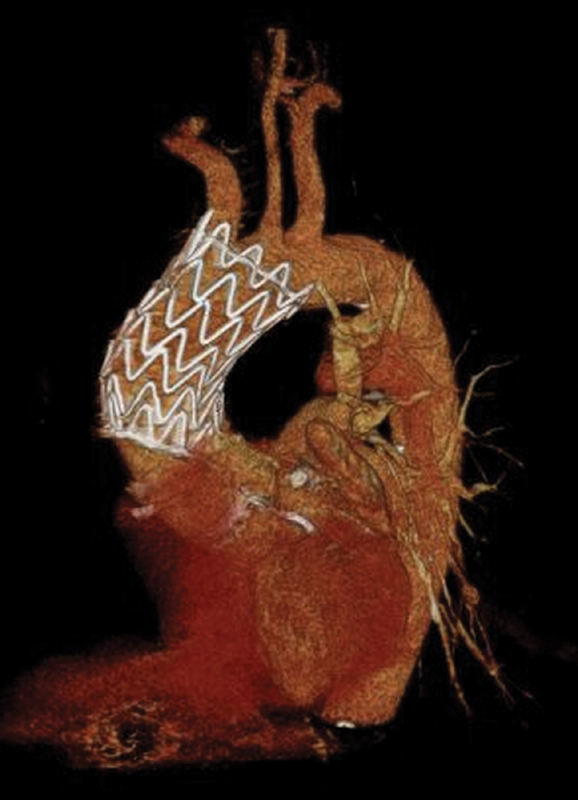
Computed tomography angiogram three-dimensional (3D) reconstruction at 8 months after endovascular repair.
